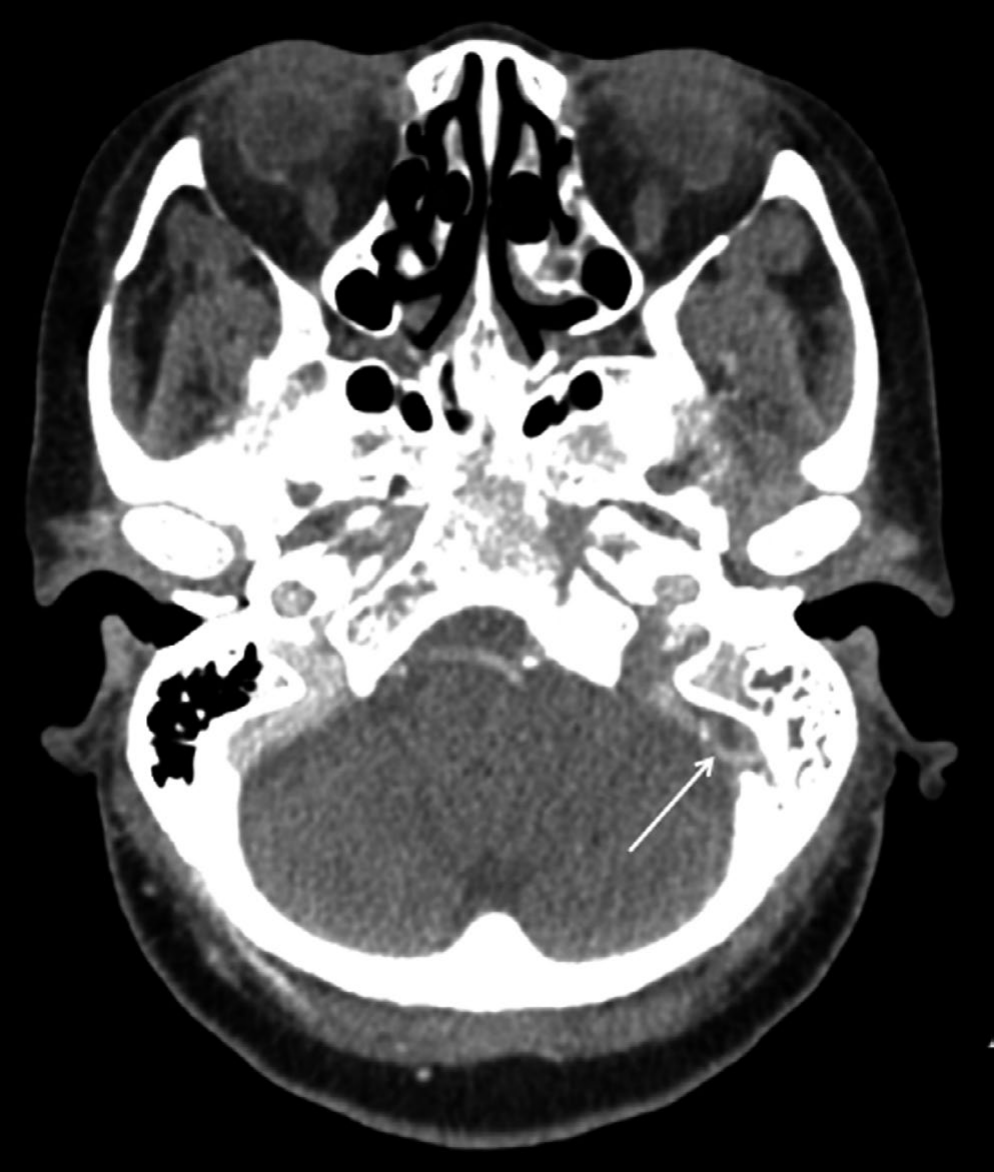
Computed tomography (CT) venography of the intracranial vasculature showing no flow in left sigmoid‐jugular complex (open arrow) in keeping with occlusive cerebral venous sinus thrombosis
